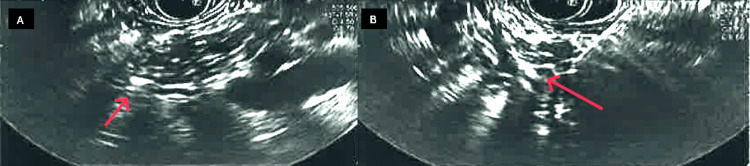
EUS images The red arrows reveal hyperechoic strands suggestive of chronic pancreatitis EUS: Endoscopic ultrasound
